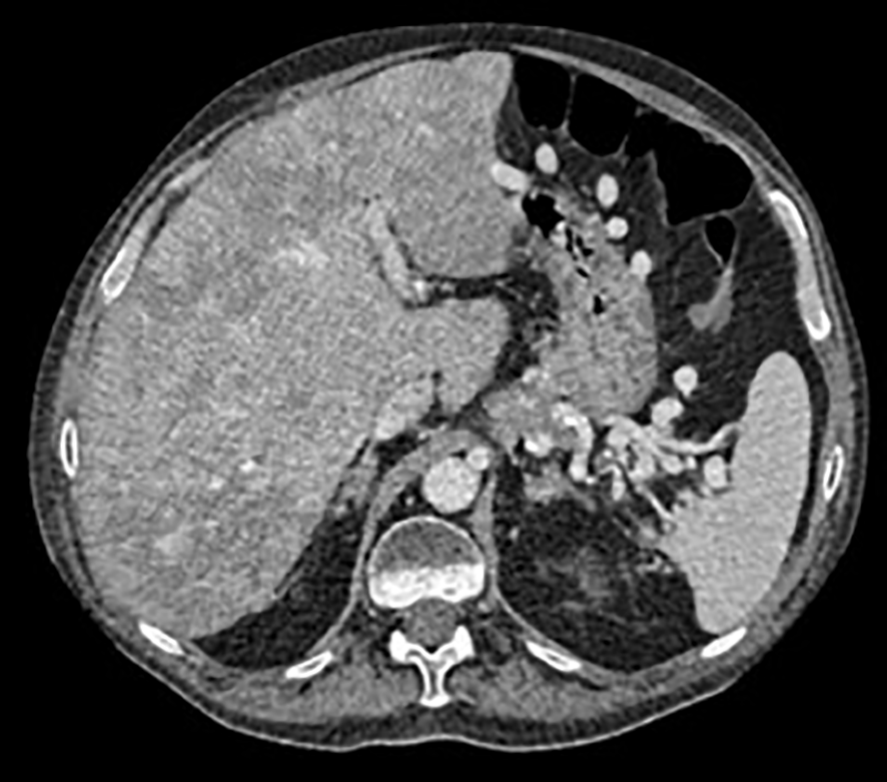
Abdominal CT scan performed 6 months after the insulinoma diagnosis showing progression of hepatic disease, with innumerable confluent focal lesions involving the entire parenchyma.
